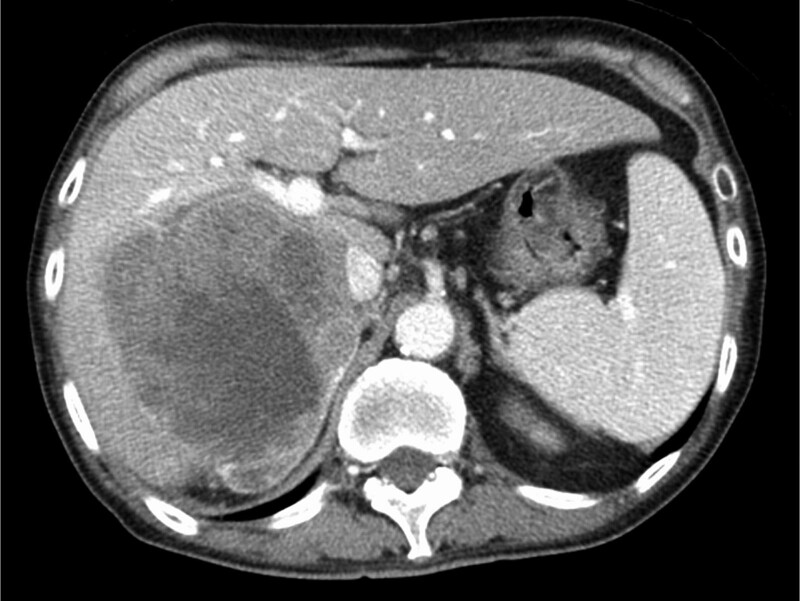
Abdominal computed tomography showing large hepatocellular carcinoma before atezolizumab plus bevacizumab treatment.
